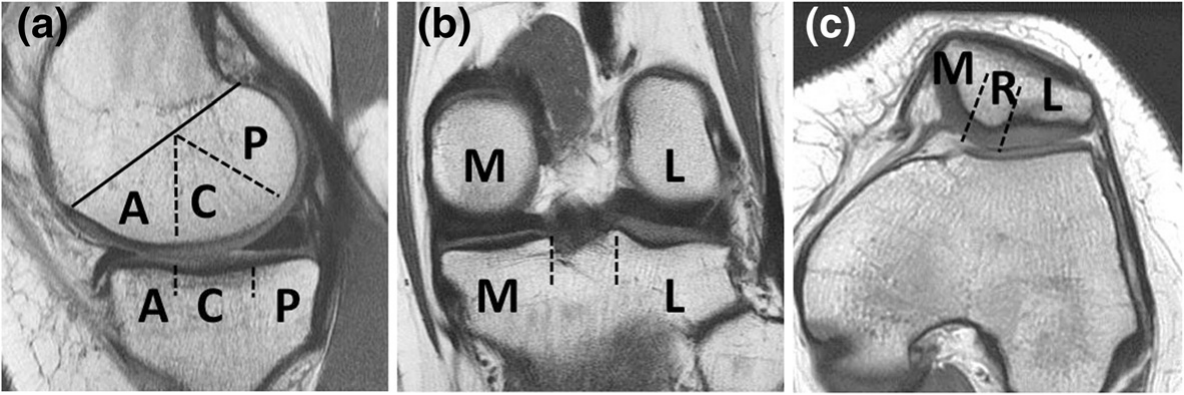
Regional subdivision of the articular surface on (a) sagittal, (b) coronal, and (c) axial MR images. A: anterior, C: central, P: posterior, M: medial, L: lateral, and R: ridge of patella, respectively.
